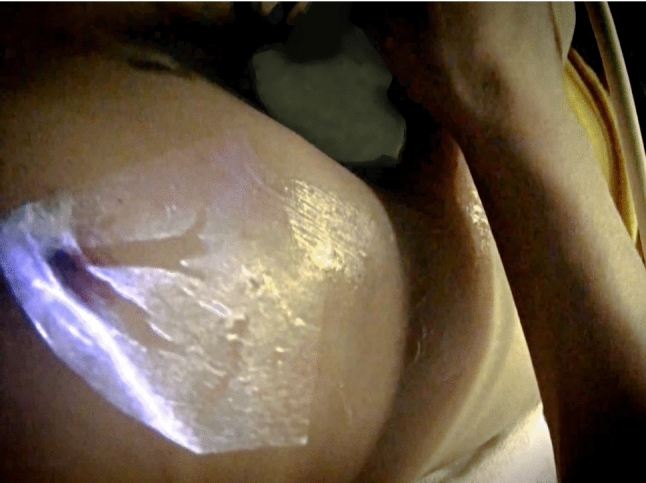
Ultrasound image overlaid onto the abdomen, captured through the HMD
